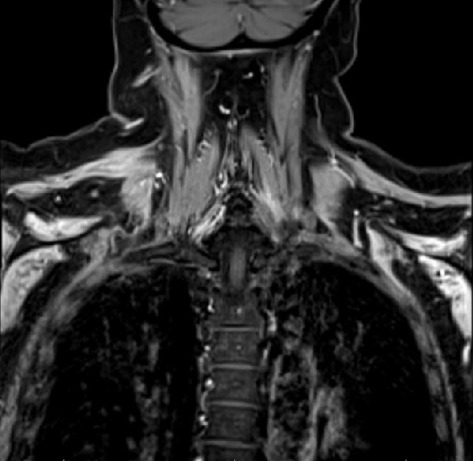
T1 MRI of the brachial plexus (limited by body habitus and movement) reveals diffuse atrophy of the shoulder musculature and homogeneous mild increased enhancement in the shoulder musculature bilaterally.
